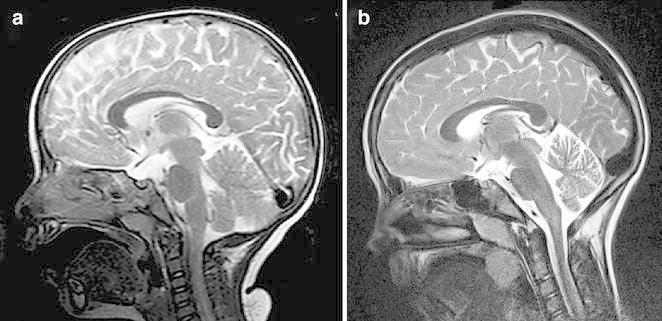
Sagittal T2-weighted cranial magnetic resonance images at onset of opsoclonus-myoclonus syndrome (a) and 5 years later (b), showing mild cerebellar atrophy with widened sulci and extracerebellar spaces
